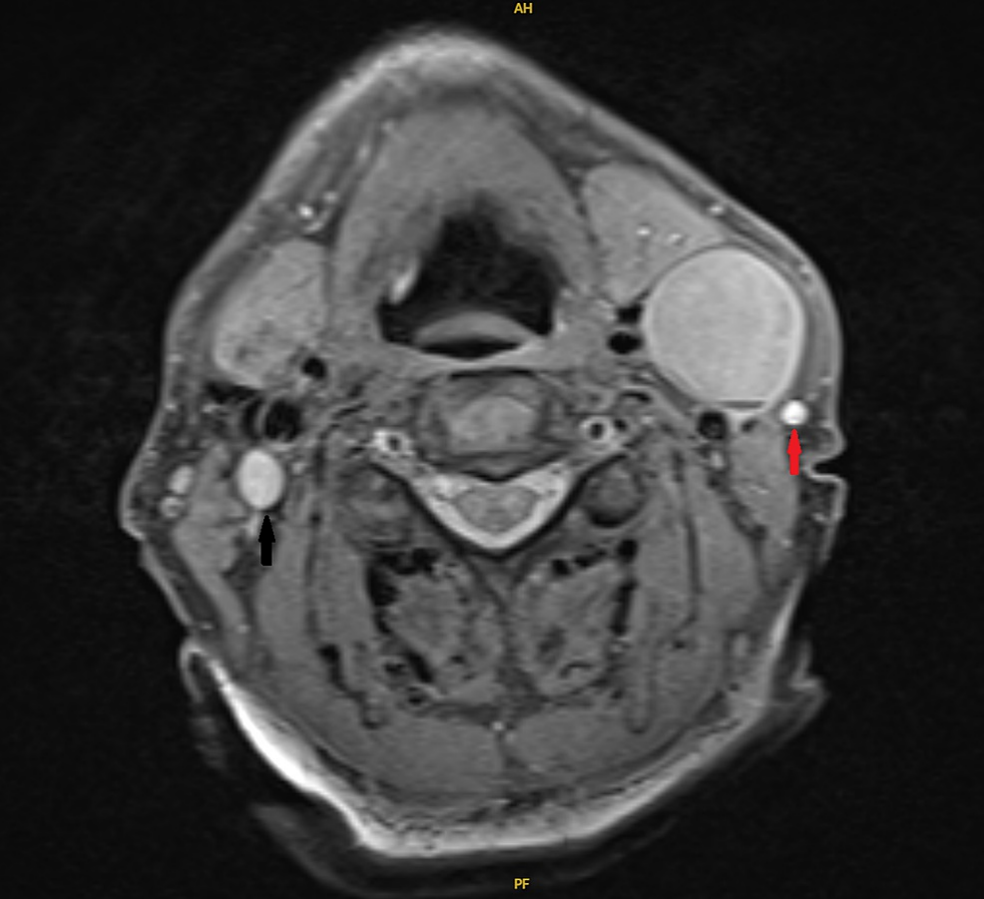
Contrast-enhanced MRI. A contrast-enhanced MRI, axial view, showing completely non-visualized left IJV that is compressed by the mass. The right IJV (black arrow) and left external jugular vein (red arrow) can be seen. IJV, internal jugular vein.
